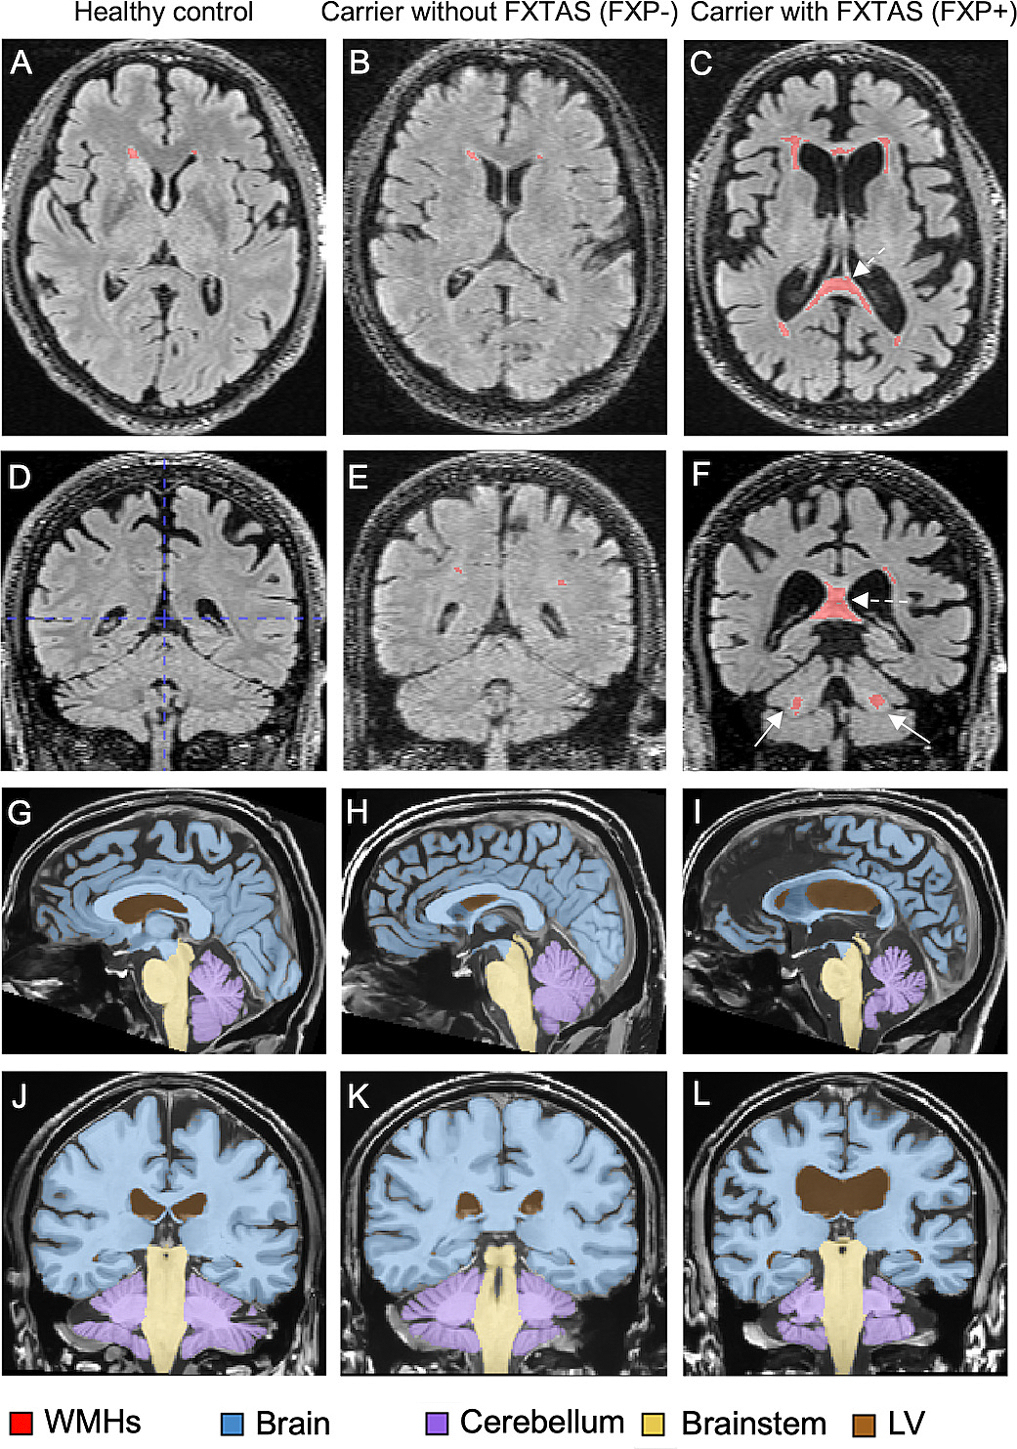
Representative segmentations of white matter hyperintensities (A–F), whole brain (G–L), cerebellum (G–L), brainstem (G–L), and lateral ventricles (LV, G–L) in a healthy control (A, D, G, & J), a premutation carrier without FXTAS (B, E, H, & K), and a premutation carrier with FXTAS (C, F, I, & L) all at the age of 62 years. (A–C) Axial views of the FLAIR scans showing the segmented white matter hyperintensities including the splenium sign of the corpus callosum (dash arrow) in C. (D–F) Coronal views of the FLAIR scans showing the segmented white matter hyperintensities including the splenium sign of the corpus callosum (dash arrow) and the MCP sign (straight arrows) in F. (G–I) Sagittal views of the T1 images showing the segmentation of the brain, cerebellum, brainstem, and LV. (J–L) Coronal views of the T1 images showing the segmentation of the brain, cerebellum, brainstem, and LV.
